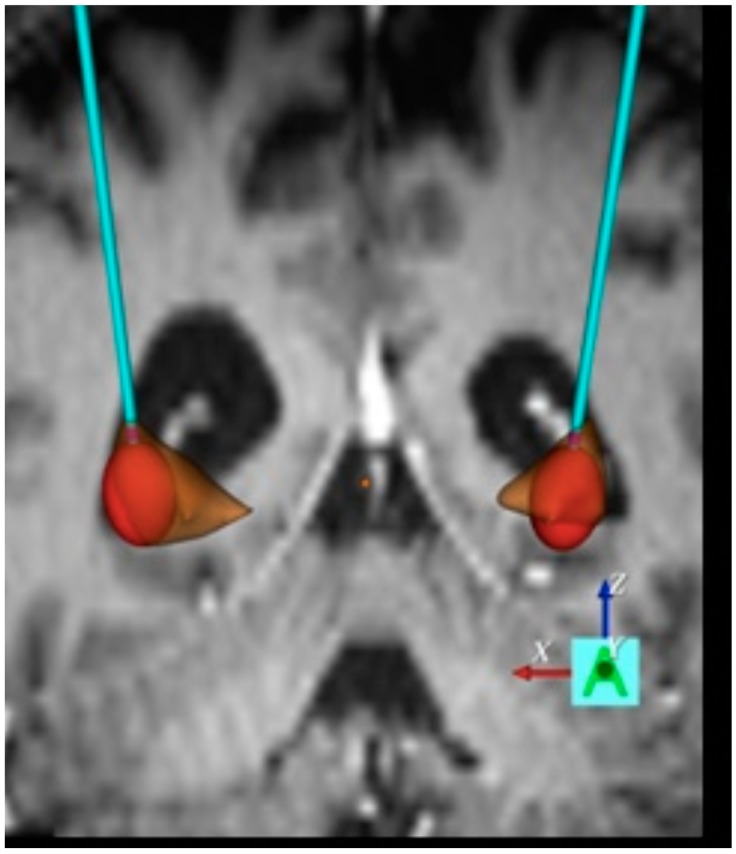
Stimulated target. Example visualization on 3D coronary MRI-view of individual electrodes and volume of tissue activated (VTA, in red) in relation to the pallidum (in brown). Image source: authors’ own contribution.
